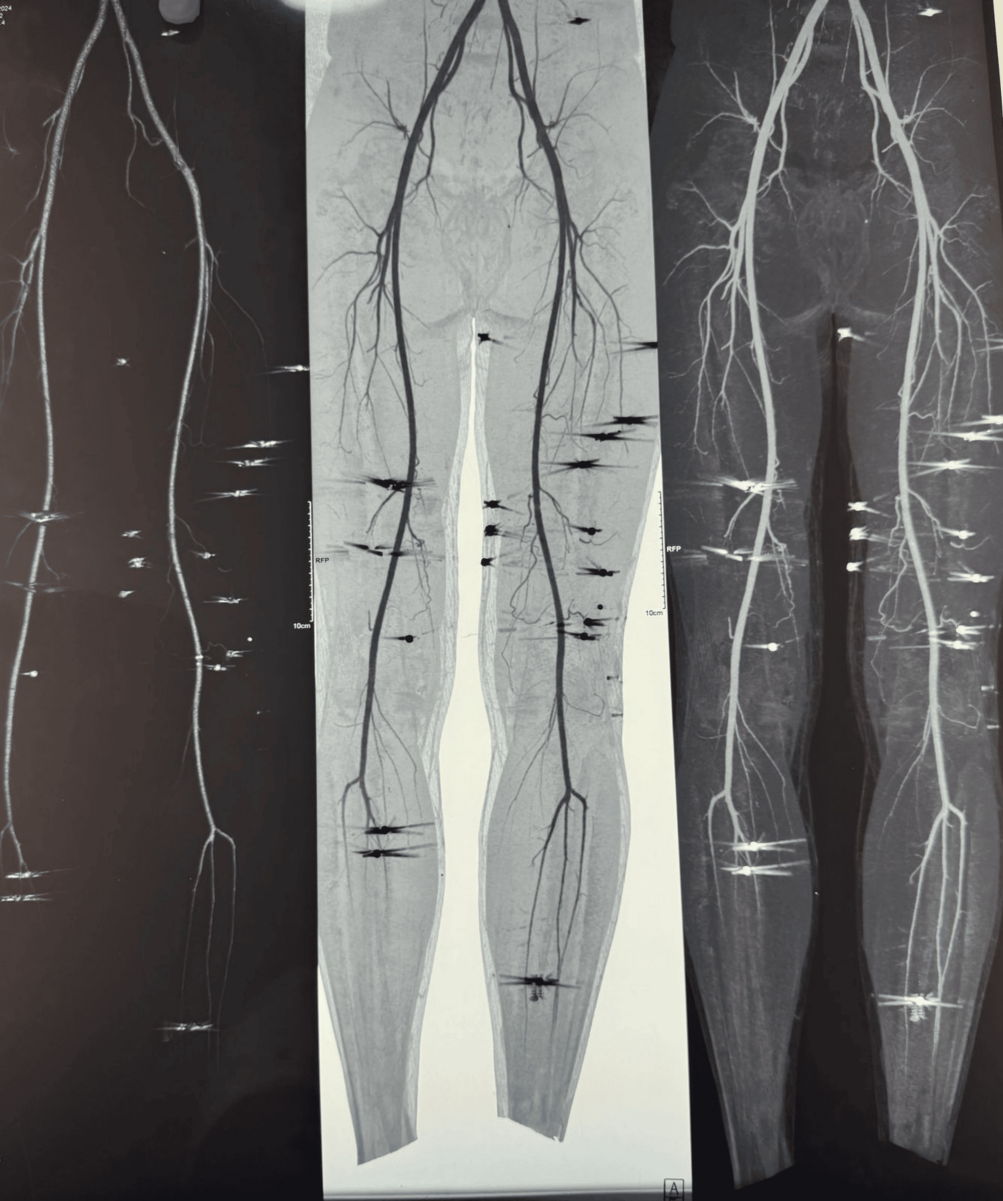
CT angiography of the bilateral lower limb arteries showing multiple tiny pellets giving streak artifacts seen in the subcutaneous and intramuscular planes of the left and right thigh and leg regions with no associated vascular injury
